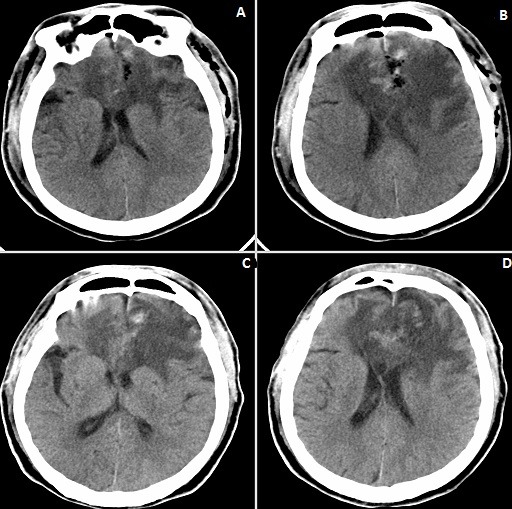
(A-D) are postoperative CT-Scans: A & B were take on postoperative day one (1) while C & D were taken on postoperative day six (6)
